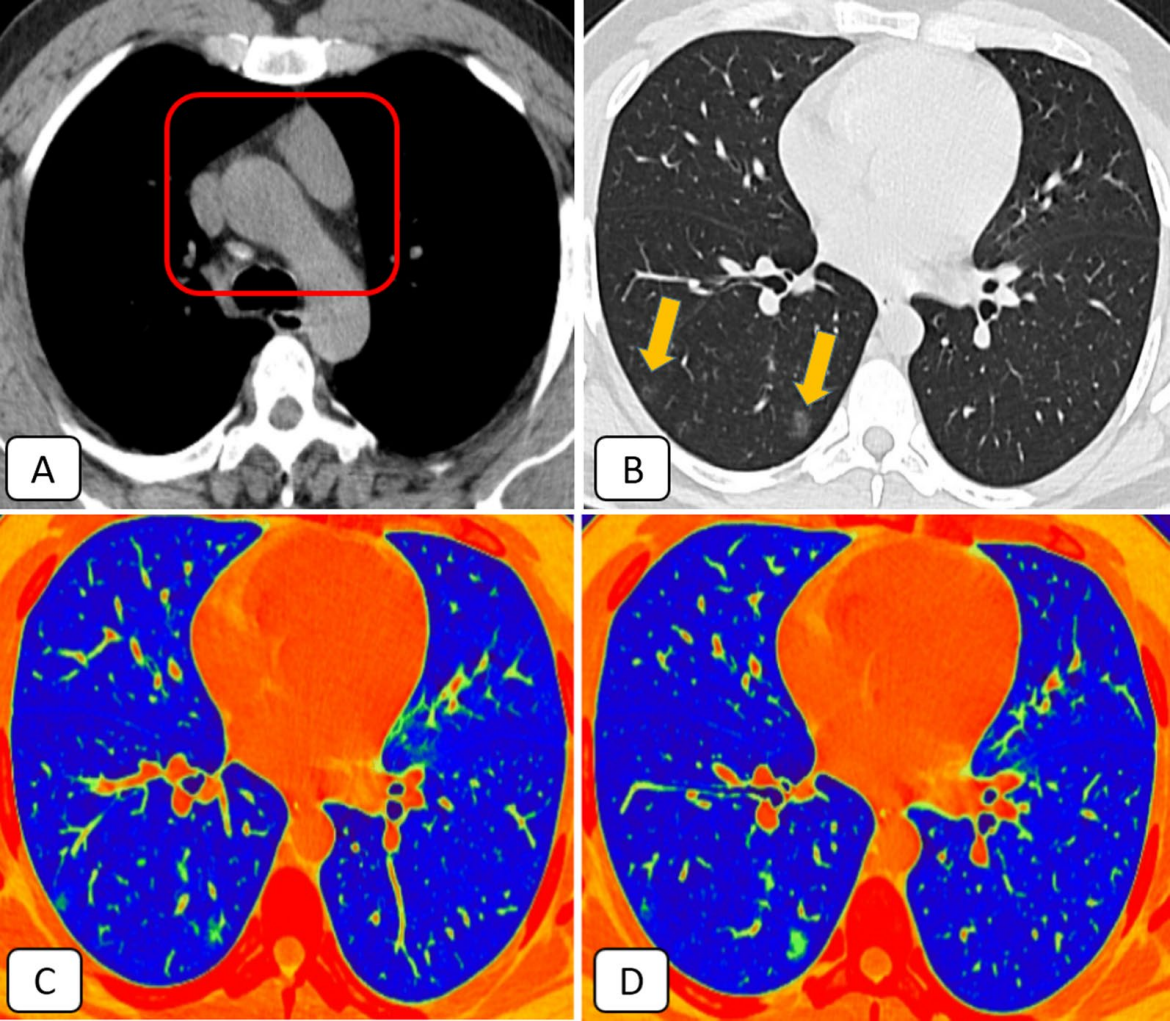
A 53-year-old male COVID-19 patient complained of fever, cough, loss of taste and smell: A Axial mediastinal window CT showed mixed soft tissue and fatty replacement of the thymic gland. The solid component is > 50% (grade 1) [red square]. B Axial lung window CT showed two right basal sub-pleural small ground-glass nodular opacities (orange arrows). C–D Axial 3D volumetry cuts highlighted the right basal small sub-pleural ground-glass opacities. The pathological lung volume was around 0.5% of the total lung volume
